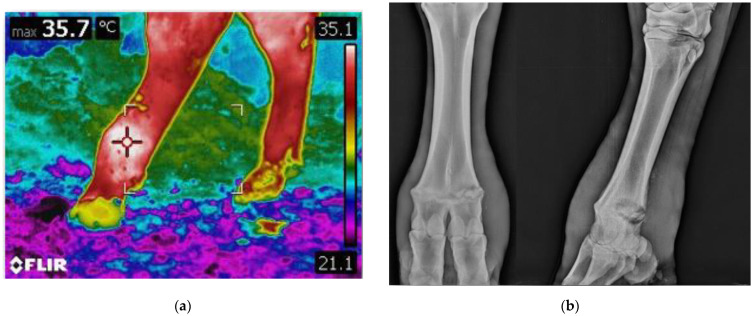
Diagnostic imaging in comparison: (a) thermographic imaging of the metatarsal region of the hind limbs, an area of increased brightness is present on the limb on the left, indicating increased skin temperature; (b) radiographic imaging of the same limb. Signs of lysis can be appreciated throughout the physis.
